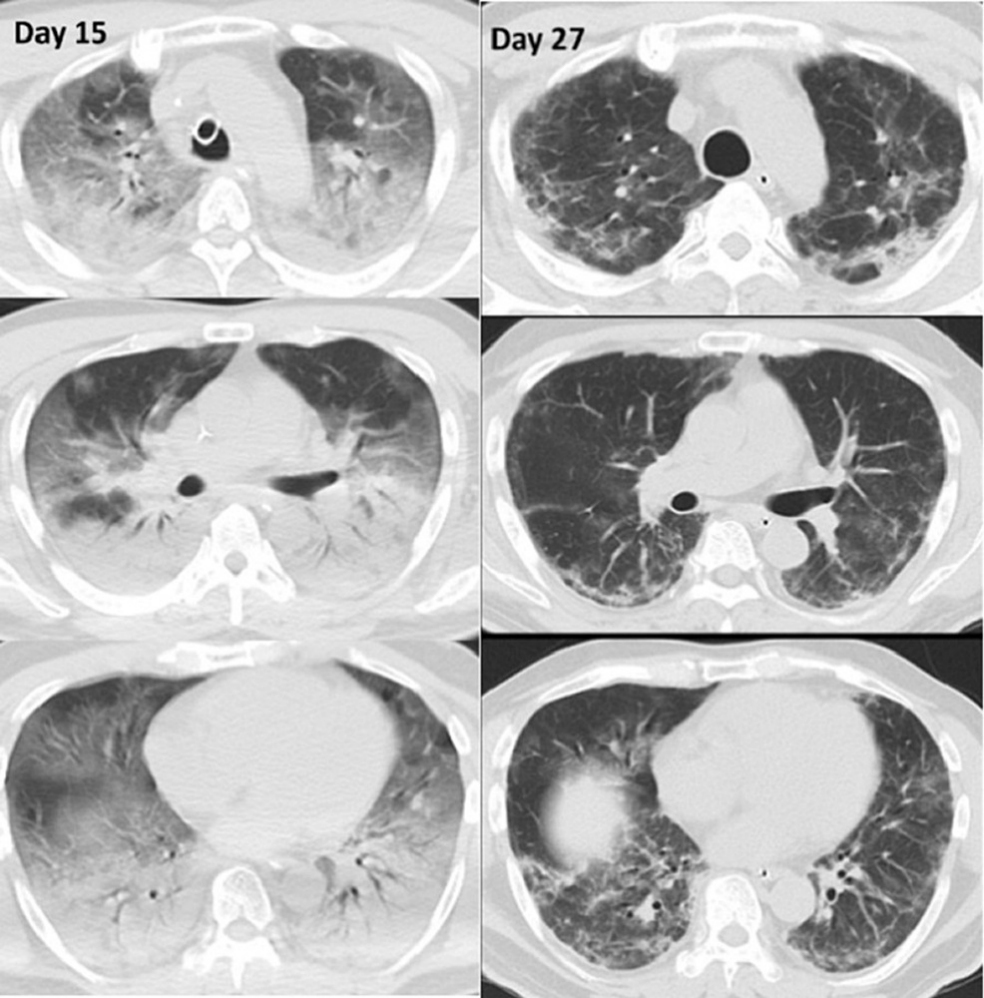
Chest CT on days 15 (left) and 27 (right). CT on day 15 demonstrates bilateral dorsal atelectasis and ventral ground glass opacities. CT on day 27 shows multiple organized lesions in the bilateral lungs.
